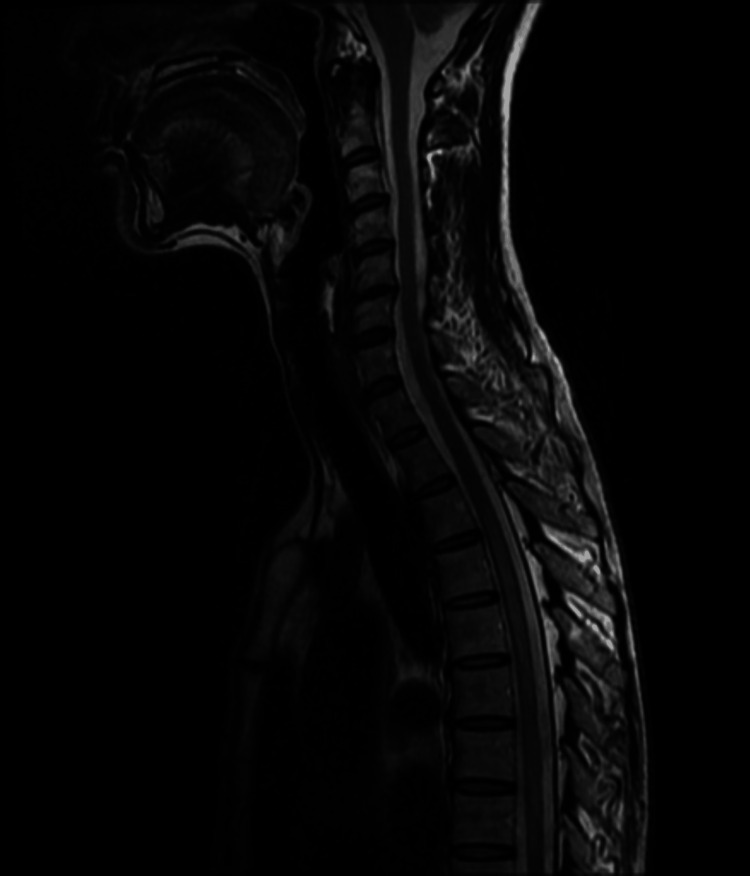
Spinal MRI at diagnosis (T2-weighted sagittal image) A longitudinal spinal cord lesion extending below the third thoracic level was observed.
